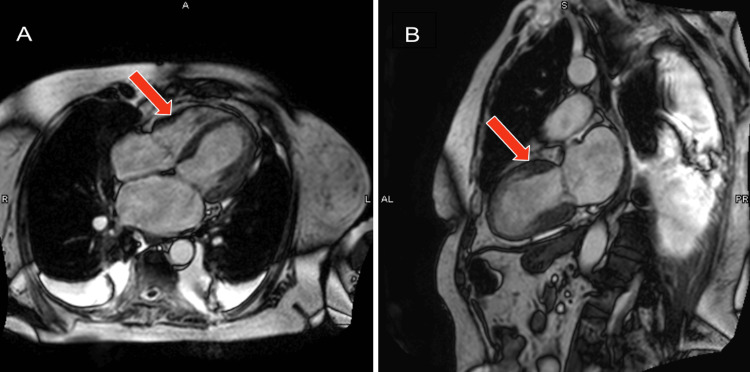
(A and B) SSFP MR images in the four-chamber and two-chamber views during peak systole showing reduced contraction of the apical segments. SSFP: Steady-state free precession; MR: magnetic resonance
